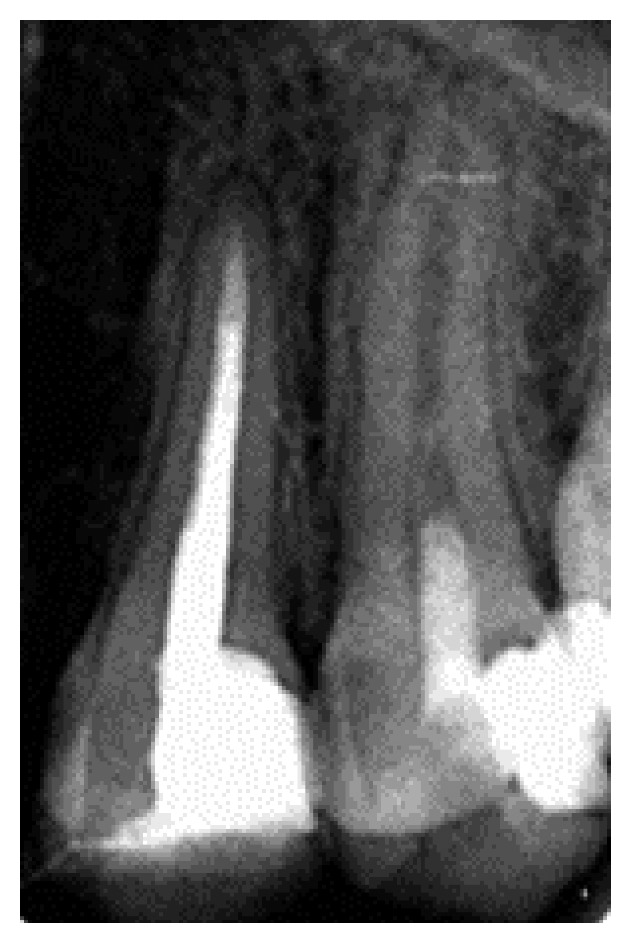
Twelve-month follow-up radiograph showing root wall thickening.
